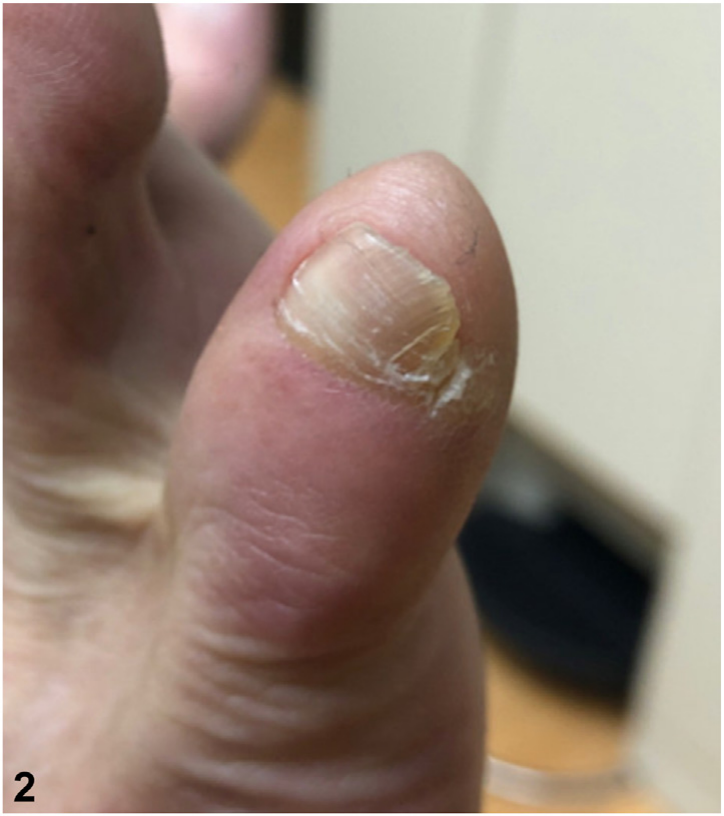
B.Subungual exostosis – Incorrect. Subungual exostosis is a benign bony tumor arising from the distal phalanx and is more common in toenails than fingernails. This presents as a nodule of the distal nail bed with accompanying onycholysis,^4^ not a nail plate split. Radiographs are confirmatory.C.Periungual fibroma – Incorrect. Also known as Koenen’s tumors in the setting of tuberous sclerosis, this is a benign papule with hyperkeratotic tip commonly presenting at the proximal nail fold.^4^ This leads to longitudinal depression in the nail plate, and not a split nail.D.Subungual verruca – Incorrect. Despite his history of warts, the fifth toenails did not demonstrate clinical or dermoscopic findings to support a viral etiology.E.Onychophosis – Incorrect. Onychophosis refers to localized or diffuse hyperkeratosis at the lateral or proximal nail folds, in the space between the nail fold and nail plate, as a result of repeated minor trauma.^1^ This does not describe the nail plate abnormality.
